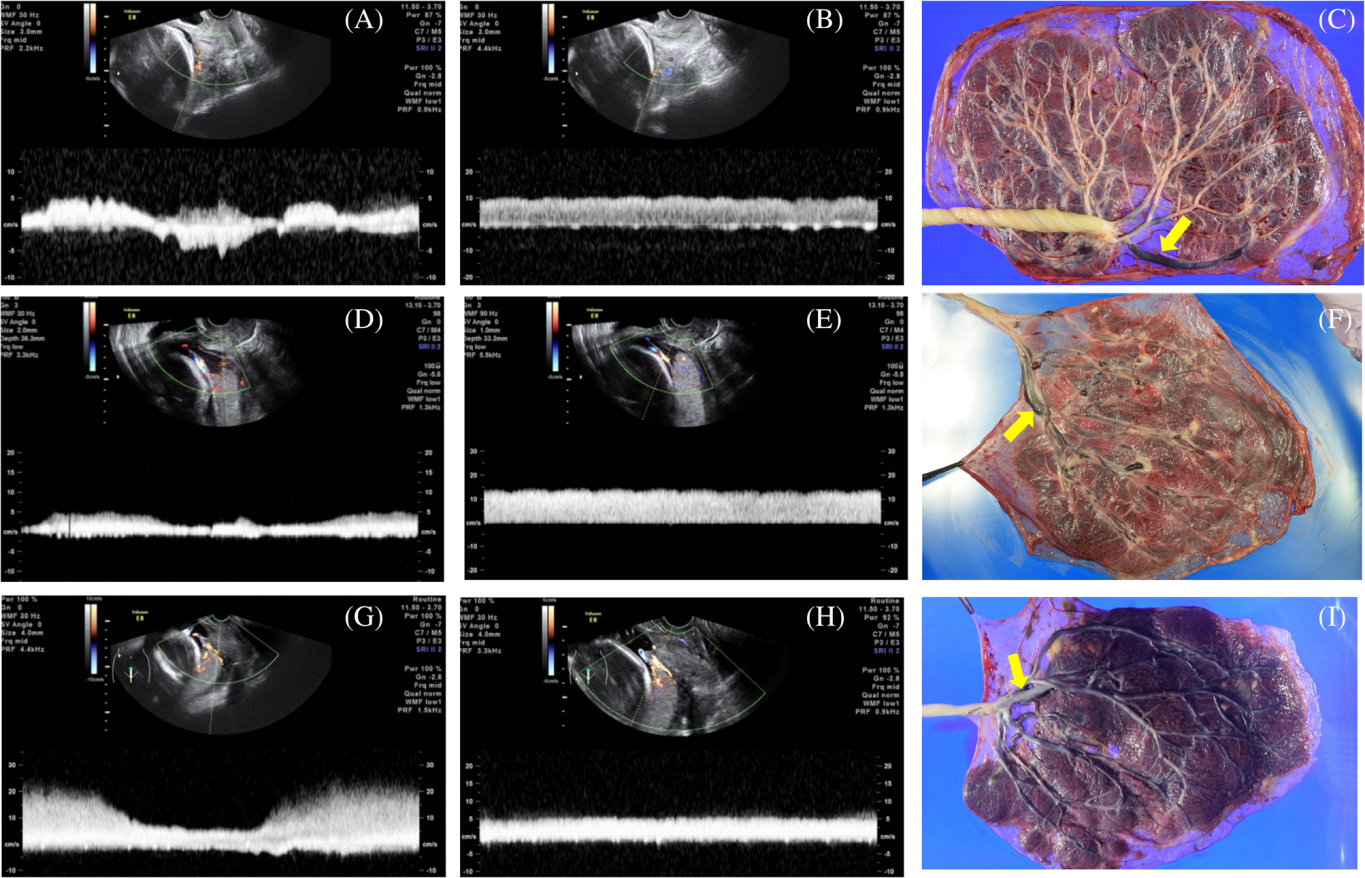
A‐C, D‐F, and G‐I show the trans‐vaginal ultrasound images and placental findings of case 1, case 2, and case 3, respectively. Fluctuation of venous flows were observed simultaneously with maternal breathing movement (A, D, G), although constant flows without maternal breathing movement were found in the fetal veins (B, E, H, respectively). In all cases, velamentous insertion of fetal veins was confirmed (Arrows in C, F, I, respectively)
